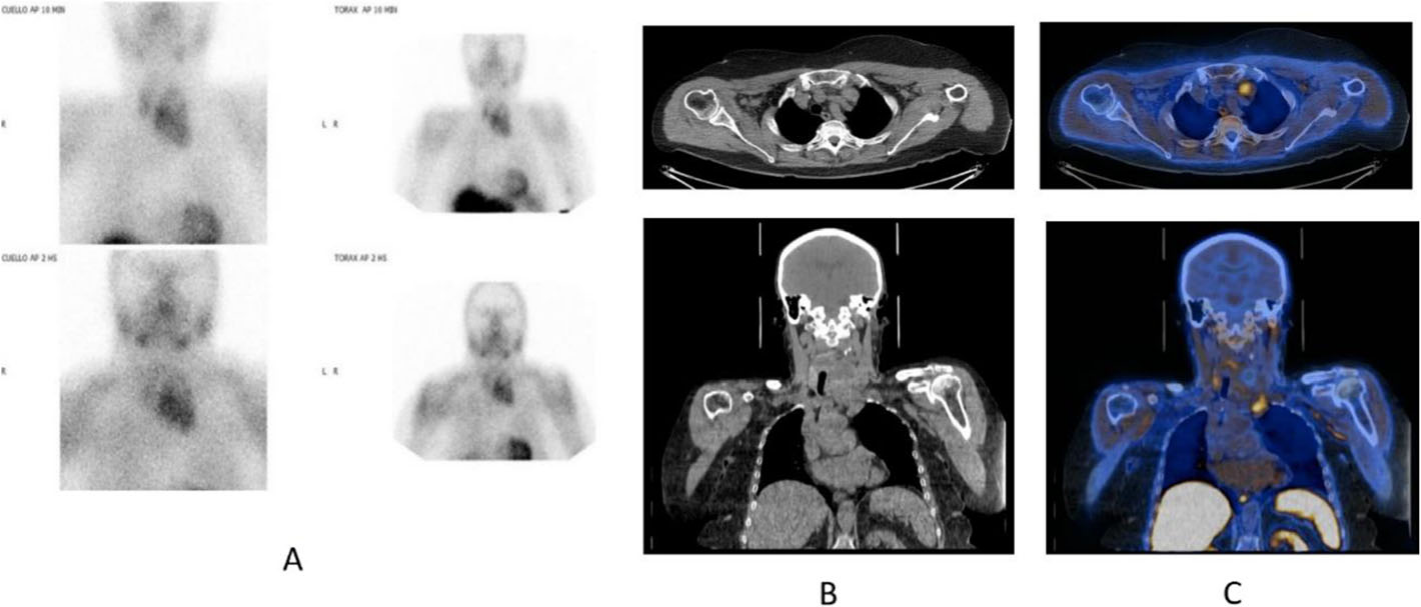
^11^C-Methionine, Primary hyperparathyroidism. *Clinical history*: 74 y.o. female patient with chronic renal failure and goitre. Parathyroid hormone 68 pg/mL. 99mTc-MIBI SPECT: multinodular goitre that extends to the thorax with a left moderate uptake nodule. There is no evidence of hyperfunctioning parathyroid tissue (a). *PET/CT findings*: multinodular goitre with a large mass in the left lobe that displaces the trachea to the right on CT (b). In the anterolateral and lower left section of this mass there is a nodule with focal tracer uptake without a clear plane of cleavage with the gland on fused images (c). These findings suggest a parathyroid adenoma
